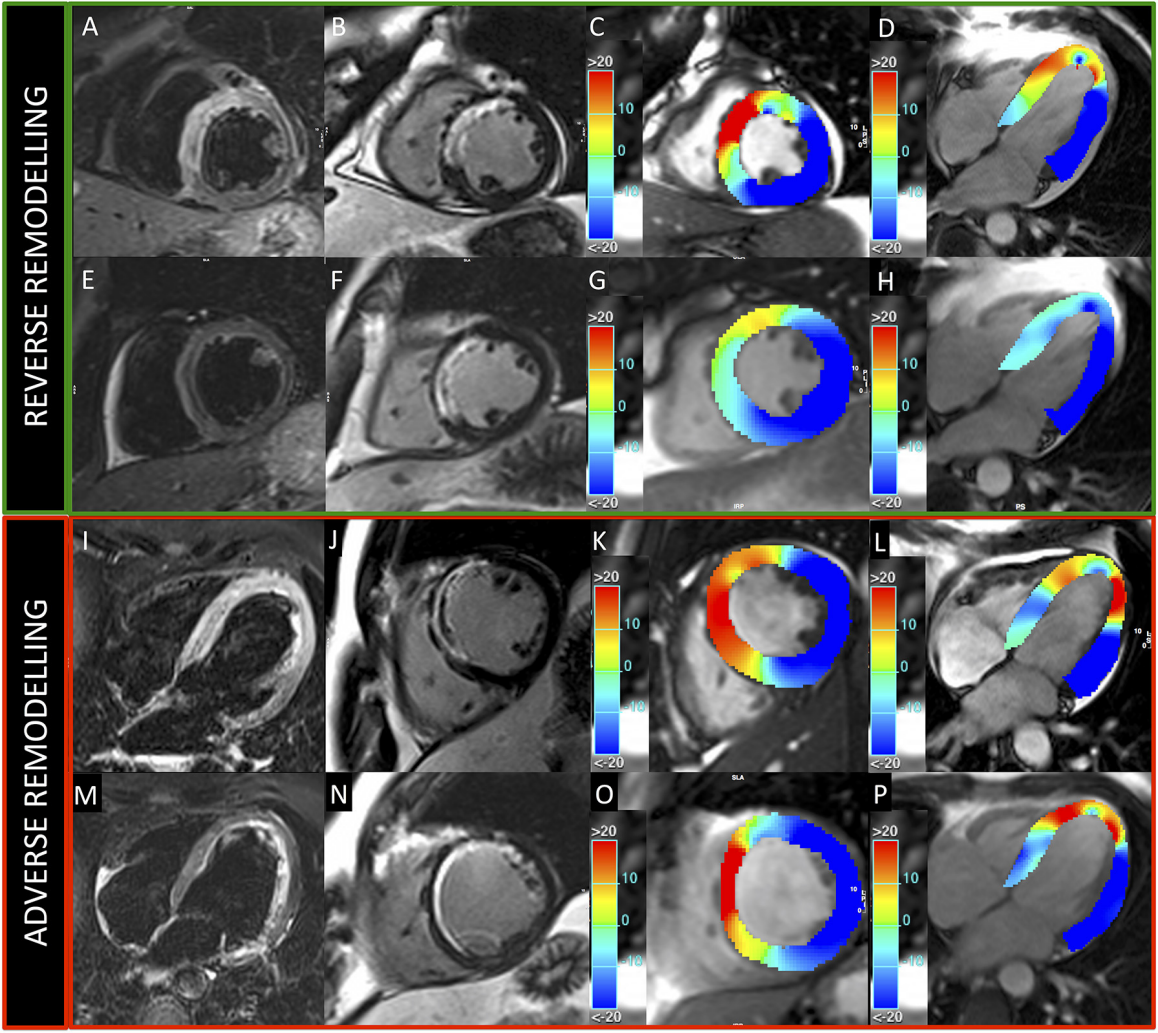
Patients presenting reverse and adverse remodeling at baseline and follow-up CMRs. Images acquired in two patients with reverse (upper green panel) and adverse (lower red panel) remodeling, treated, respectively, with 500 and 250 mg ASA dose. Upper and lower rows represent exams acquired at baseline and 6-month follow-up, respectively. T2-weighted (A,E,I,M) and LGE (B,F,J,N) images show anterior myocardial infarction in both cases. GCS (C,G,K,O) and GLS (D,H,L,P) colored maps demonstrate better baseline values and higher recovery of myocardial contractility at follow-up in the patient with reverse remodeling.
